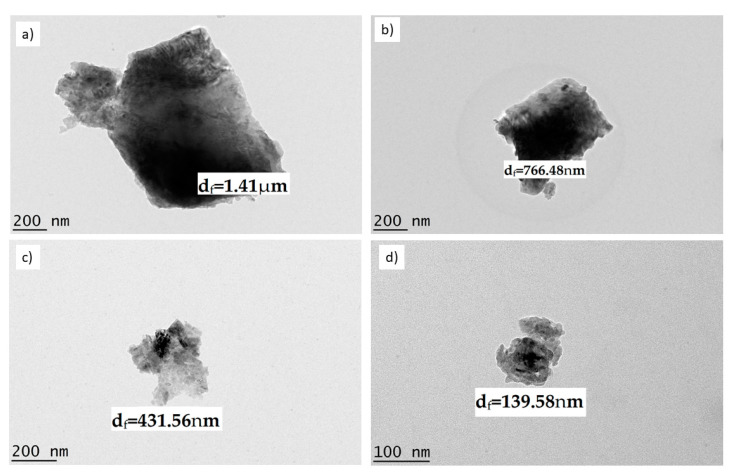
TEM images of dolomite that has been ball-milled at 500 rpm and ultrasonicated for 2 h with (a) 30% amplitude and 1 repetition (D_301X_), (b) 50% amplitude and 2 repetitions (D_502X_), (c) 50% amplitude and 3 repetitions (D_503X_), and (d) 50% amplitude and 10 repetitions (D_510X_).
